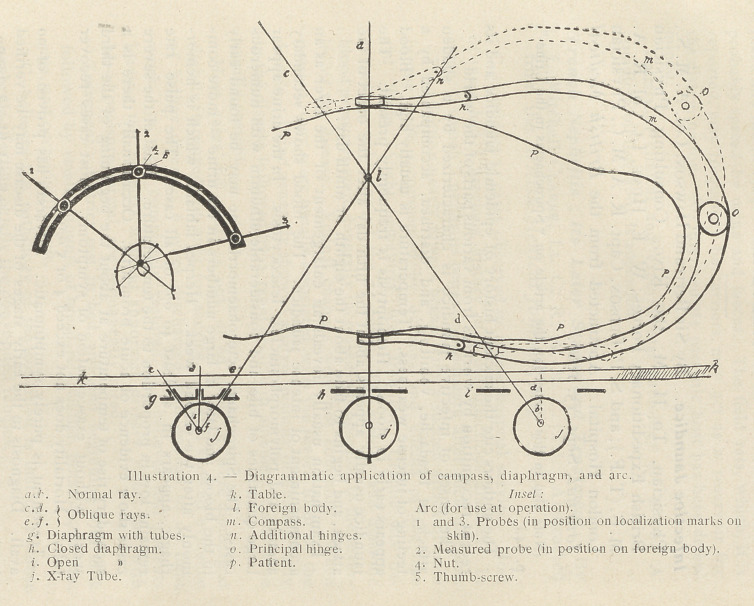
# Simplified X-Ray Methods
